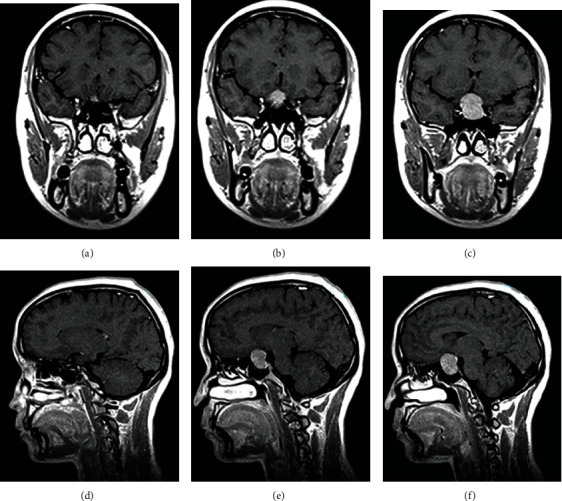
MRI finding of pituitary tumors.
